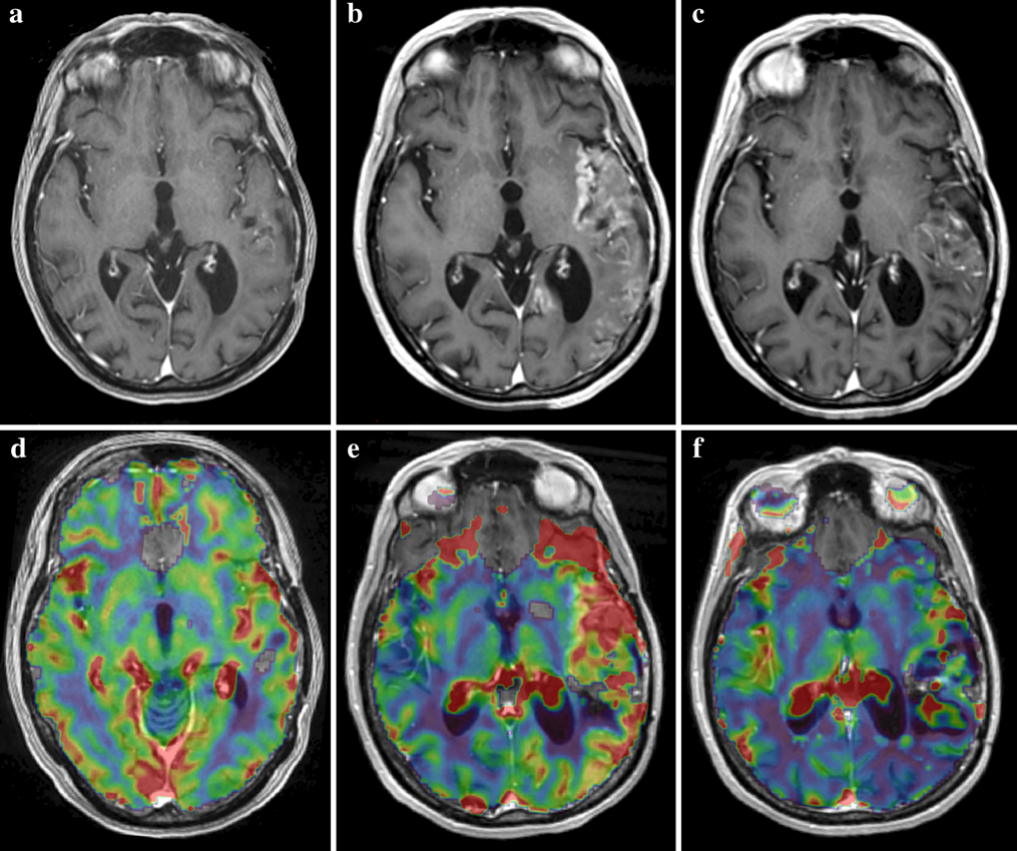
T1-weighted MRI with gadolinium a 5 months prior to admission, b during admission, c 2 months after admission, d–f DSC perfusion-weighted MRI d 5 months prior to admission, e during admission, and f 2 months after admission
